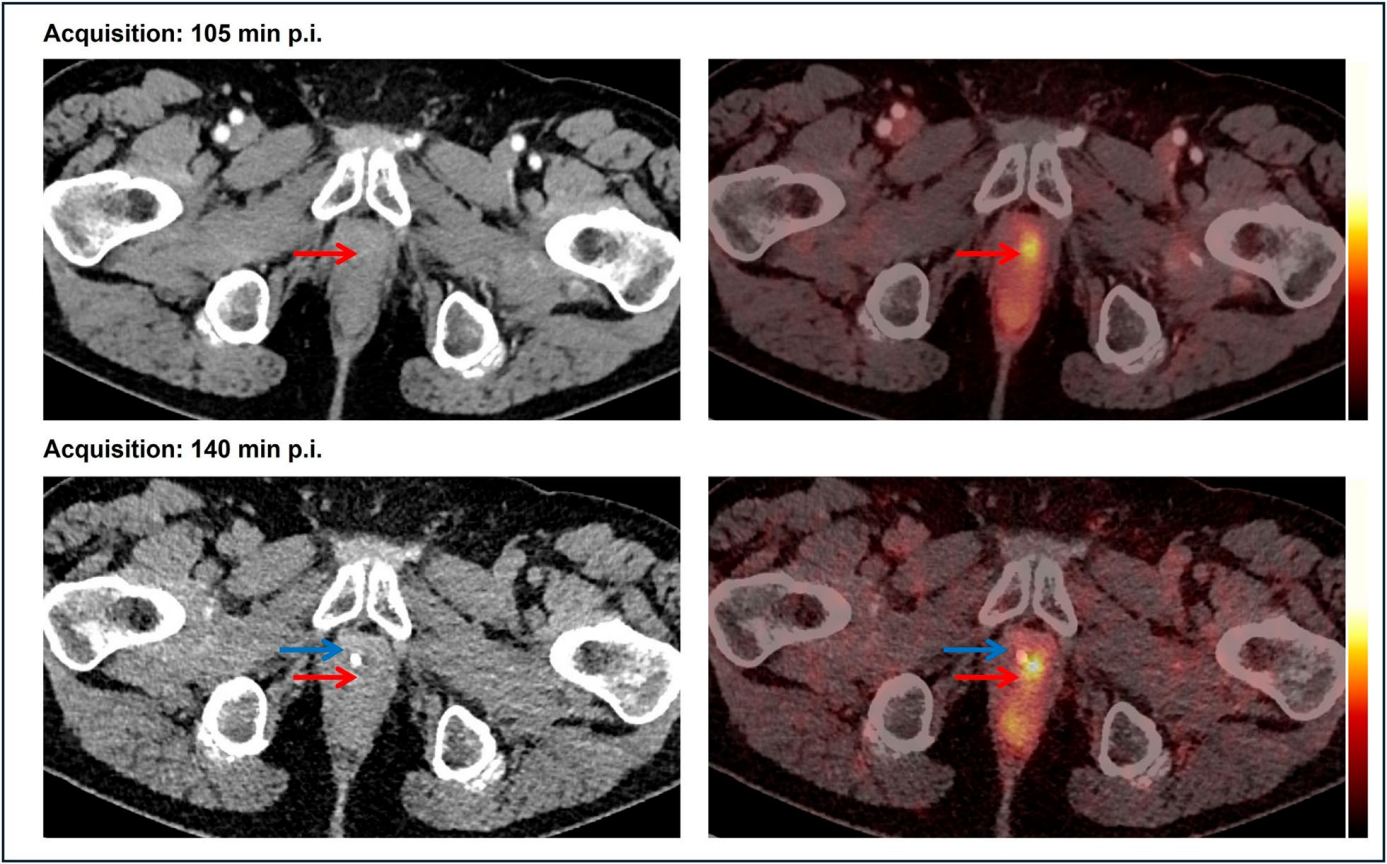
The juxtaposition of focal [^18^F]PSMA-1007 uptake to the urethra complicates the discrimination of culprit tracer uptake (red arrow) from residual radiourine in the urethra (blue arrow). In addition, the delayed image provided an increase in SUV metrics, leading to upgrade of the lesion from PSMA-RADS 3 A to PSMA-RADS 5
